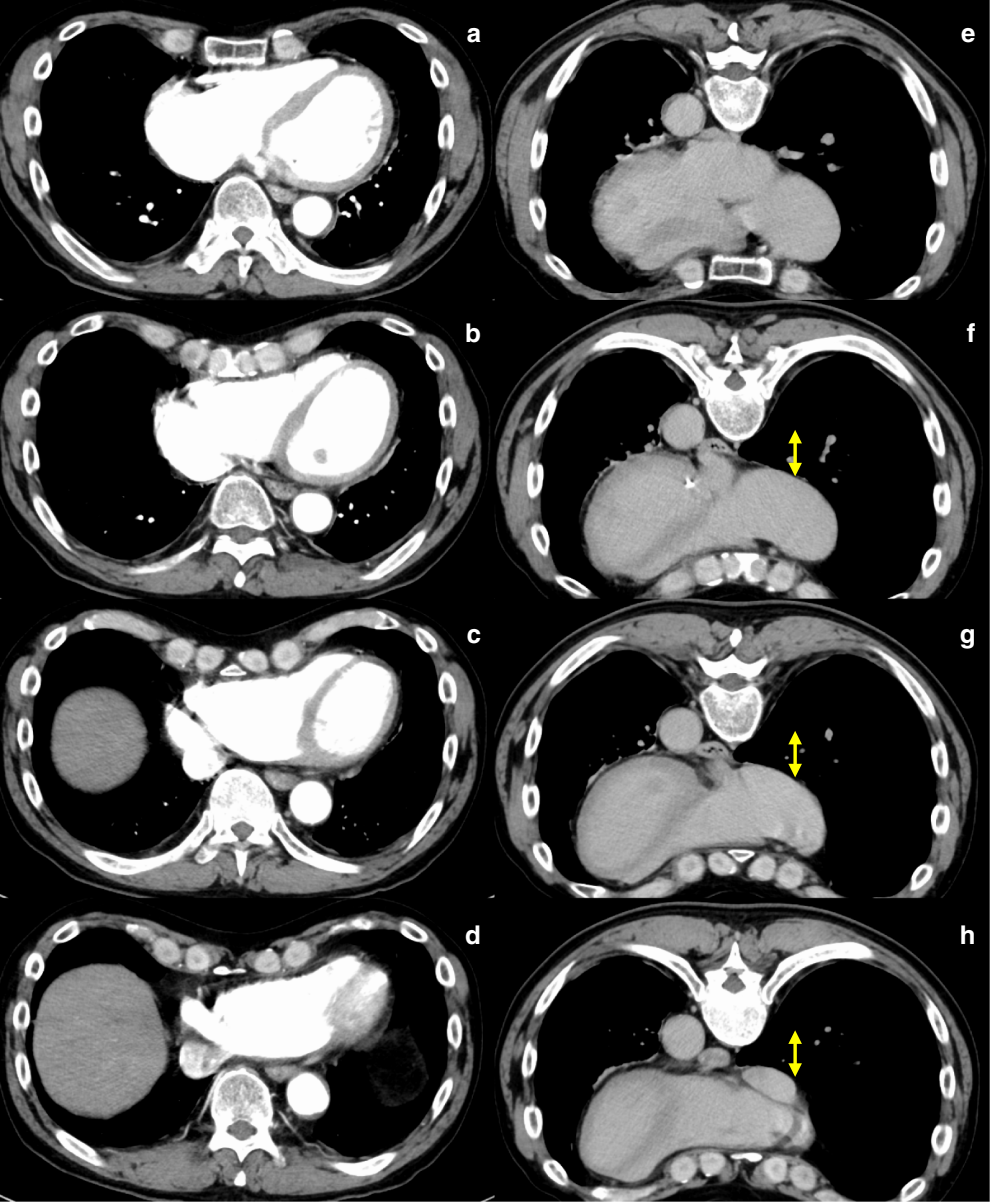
Patient 2. a–d Contrast-enhanced CT in the supine position showing the pectus excavatum. The Haller index score was 4.3. e–h CT image in the prone position revealed dilated mediastinum (arrow) compared to a–d. CT slice level was matched based on the sternal bone
